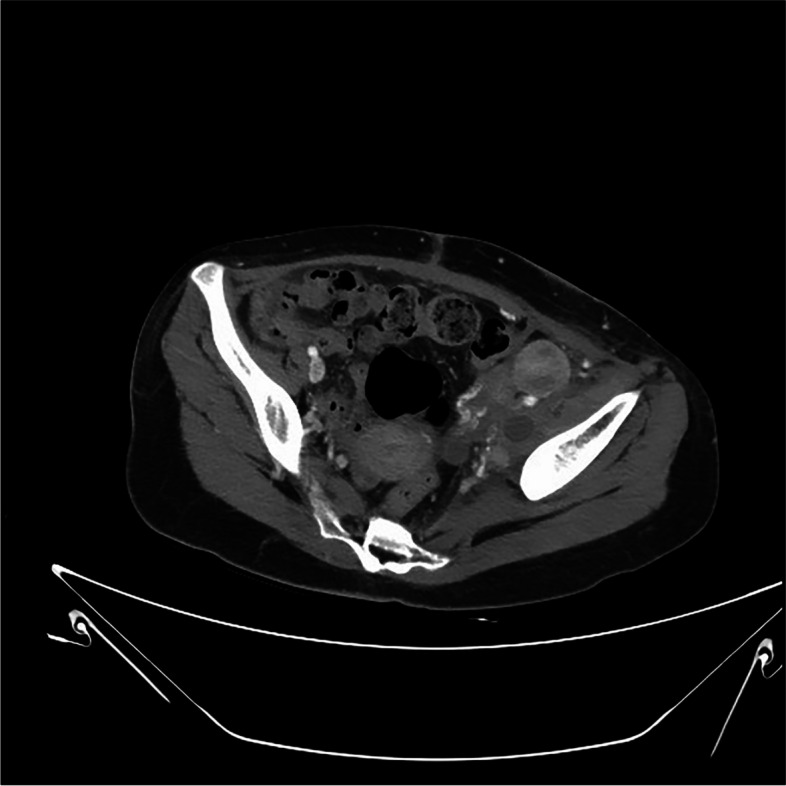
A female patients (patient number 5 in Table 2) with epithelioid sarcoma in right inguinal fold experienced tumor rupture in her retroperitoneum. This was her computerized tomography (CT) scan before rupture
